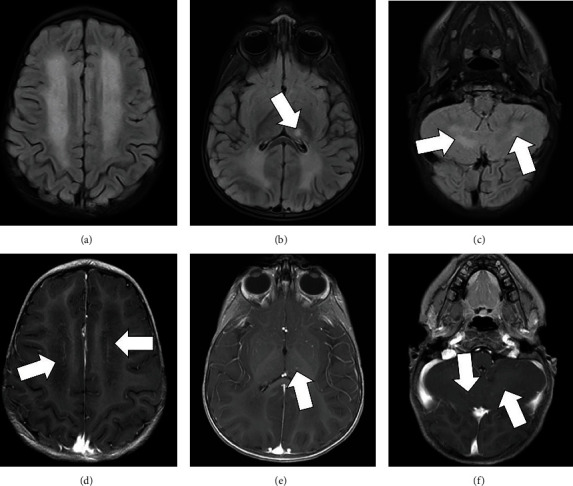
MRI obtained 10 days after admission demonstrating mild progression in diffuse white matter T2 FLAIR hyperintensity (a–c) with slightly increased involvement of the left lateral thalamus (arrow in (b)). Previously noted punctiform enhancement in the supratentorial periventricular white matter has nearly resolved with minimal residual curvilinear enhancement, possibly vessels (arrows in (d)). Small curvilinear enhancement is again seen in the left thalamus (arrow in (e)). Prior curvilinear enhancement in the cerebellum had resolved with interval development of few new punctiform cerebellar enhancing foci (arrows in (f)).
